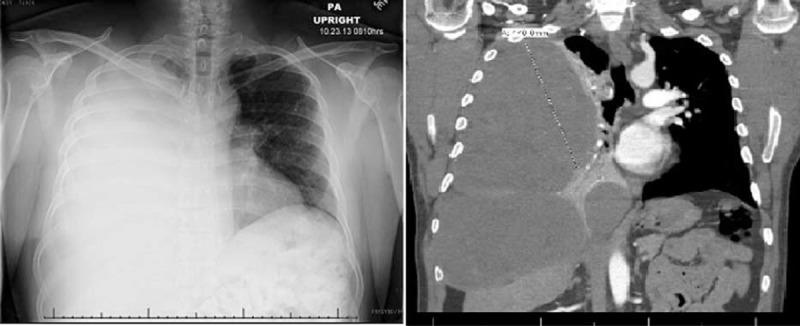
Chest x-ray and coronal view of CT scan on admission.
